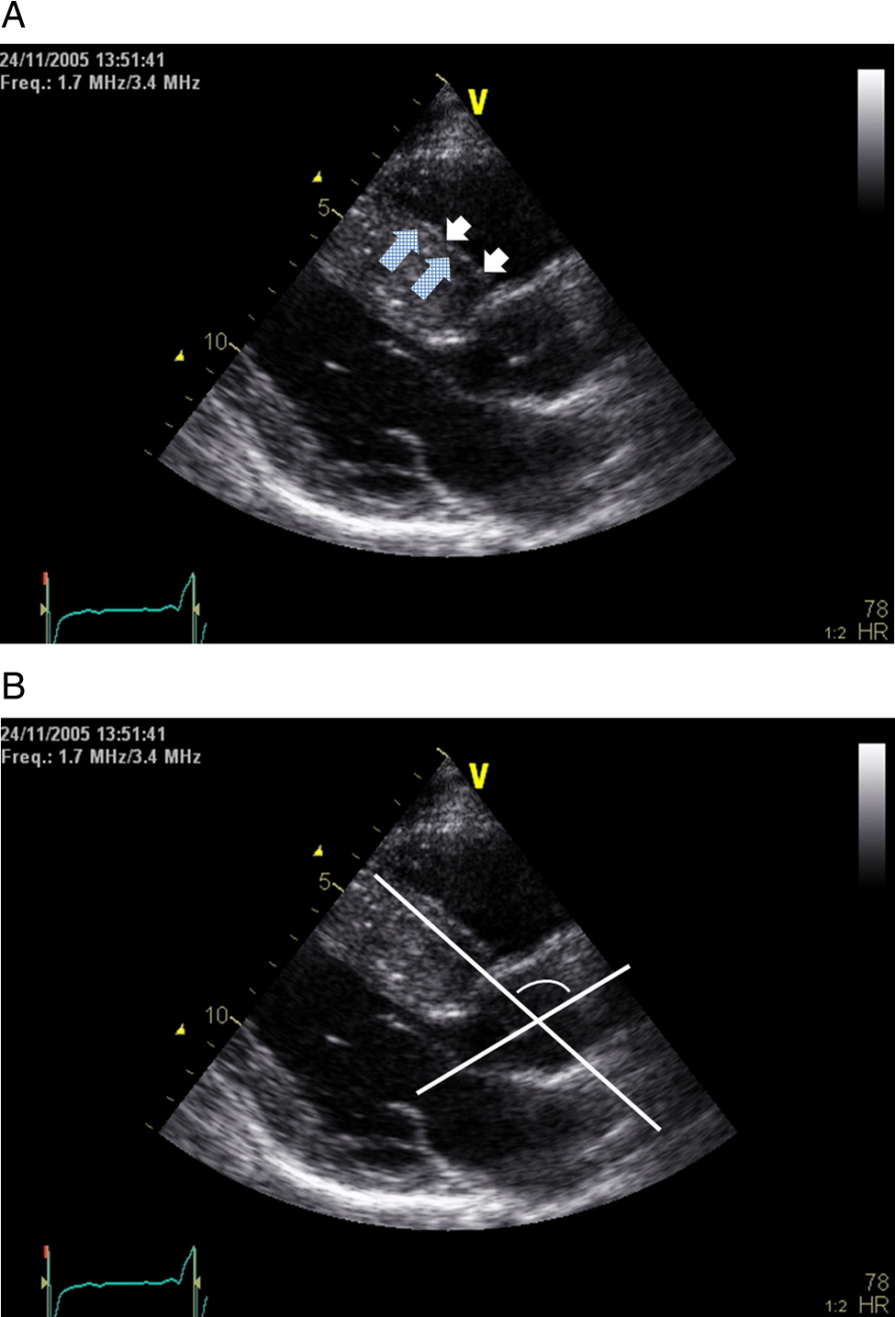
Transthoracic echocardiogram, parasternal long-axis view: example of construction of reference lines for aortoseptal angle calculation. (A) The septal line was drawn along the junction of left and right interventricular septum (checked arrows), parallel to the proximal right endocardial border (white arrows). (B) The aortoseptal angle was defined as the angle between the septal line, and a line drawn through the long axis of the aortic root where a value of 180° would be a straight line from septum to aorta and reducing values represent increasing angulation.
